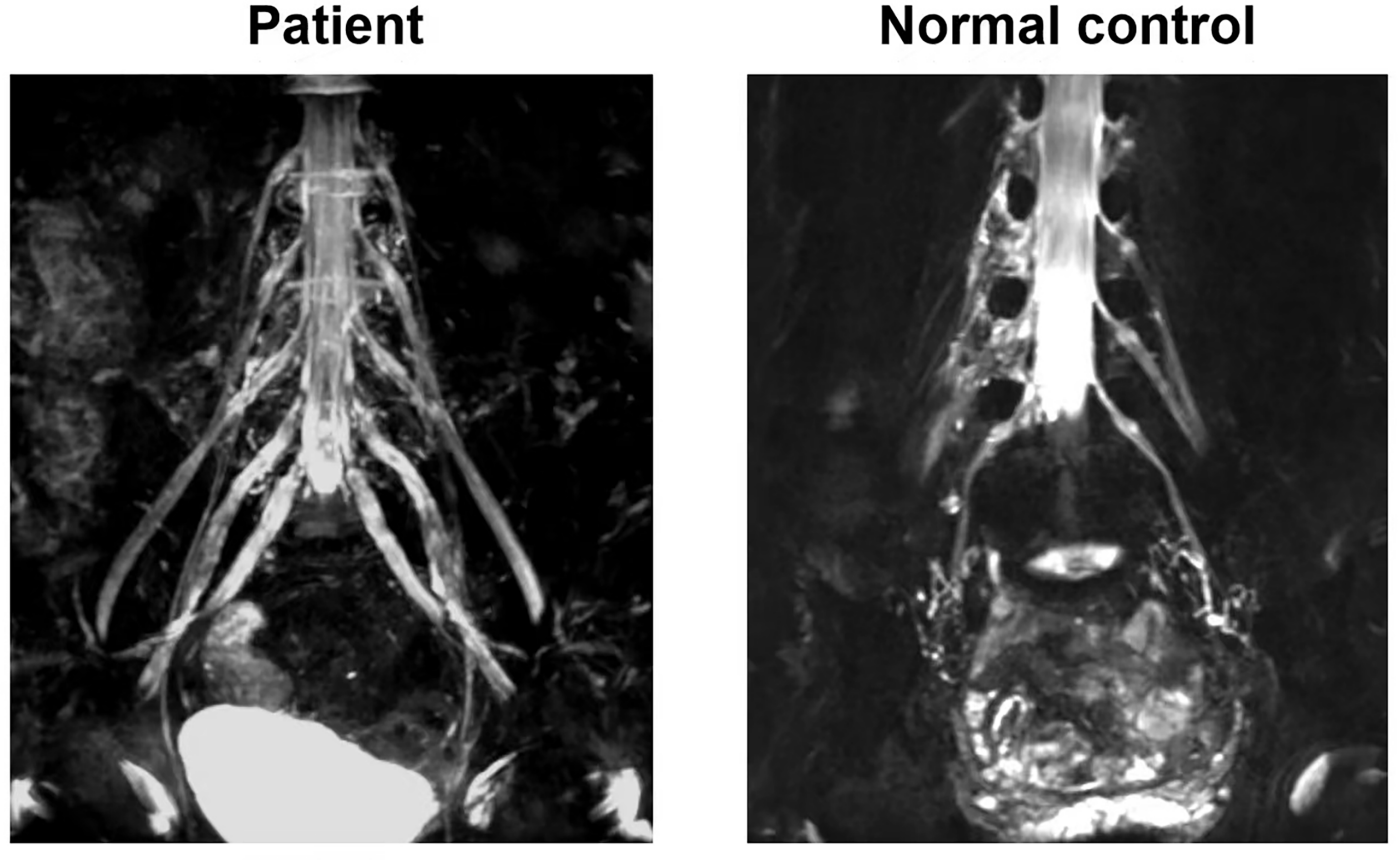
MRI neurography with enhancement imaging of lumbosacral spinal roots and plexuses. The patient showed marked symmetric hypertrophy and increased signal intensity in lumbosacral roots and plexuses comparing with normal control.
